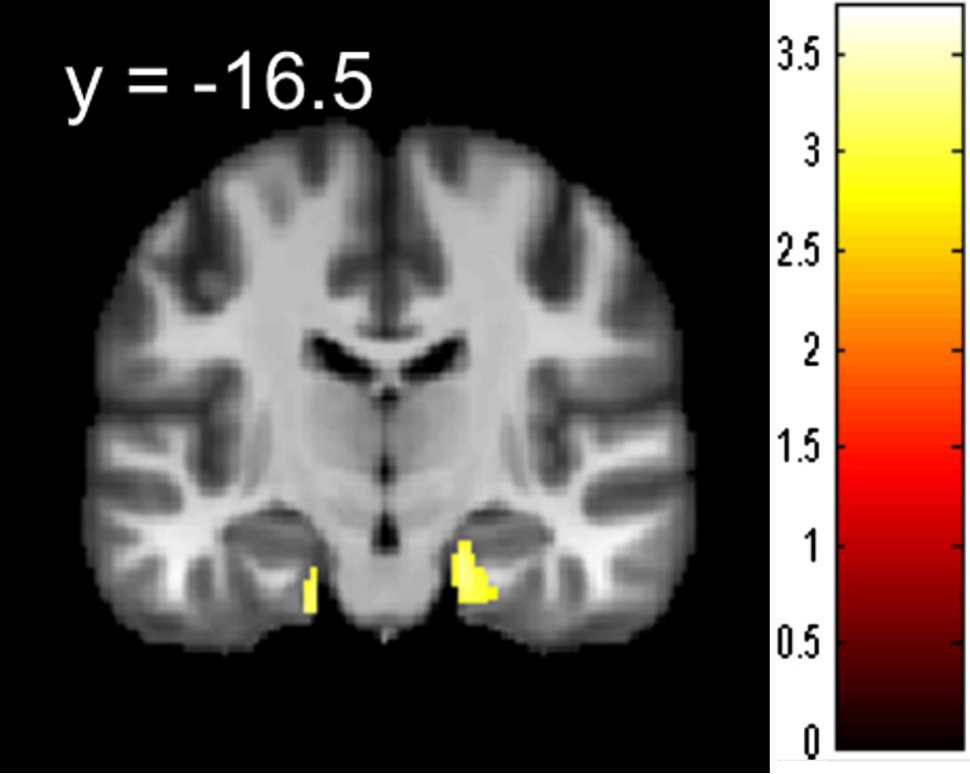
Maps showing larger rWMV in humanities students than in science students. The results are presented at a threshold of *P* < 0.0025, uncorrected, on a skull-stripped image created from the averaged normalized T1-weighted structural images of a portion of the subjects included in this study. The region of significant difference is visible in an anatomical cluster that is mainly concentrated around the right hippocampus. The intensity of the *color* represents the *T* score
